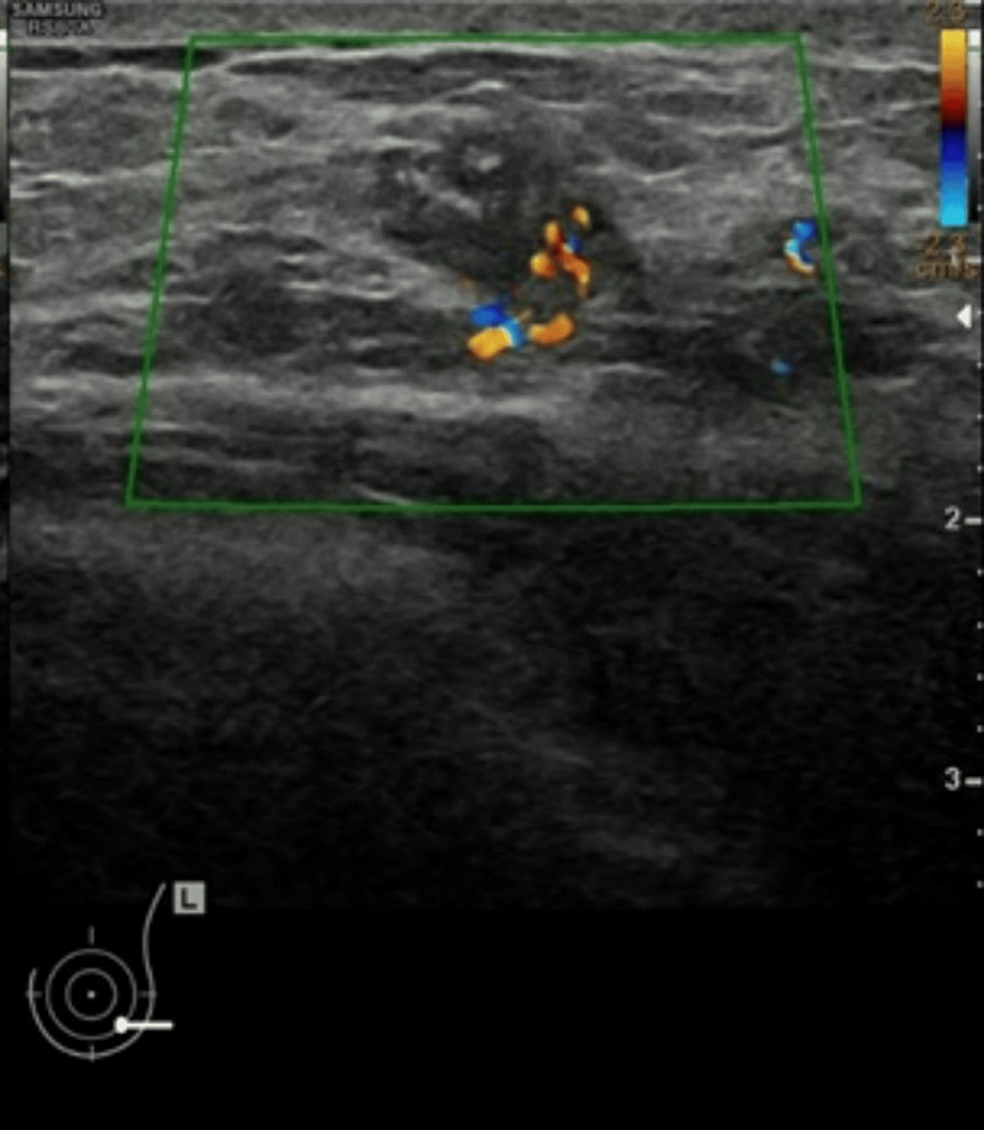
Left breast ultrasound scan revealed a 19-mm irregular suspicious lesion in the lower outer quadrant with increased vascularity (green trapezoid).
